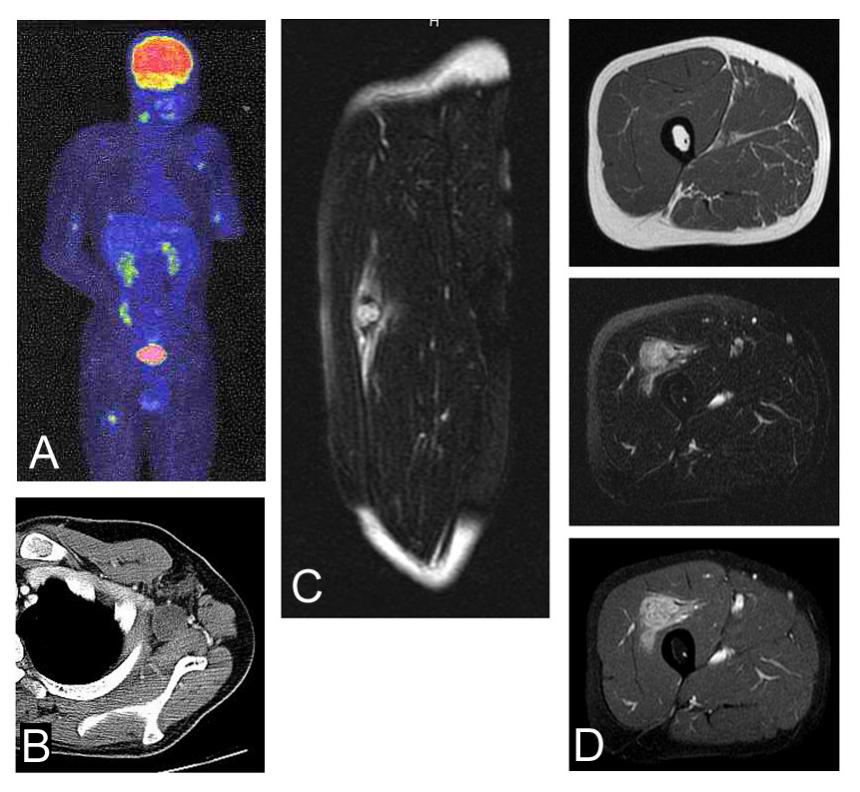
Metastatic lesions of epithelioid sarcoma. FDG-PET identifies lesions with an increased uptake in the right neck (SUVmax; 4.6), right upper arm (SUVmax; 4.1 g/ml), left upper arm (SUVmax; 4.2 g/ml), right thigh (SUVmax; 5.5 g/ml) left thigh (SUVmax; 2.0 g/ml), back (SUVmax; 3.6 g/ml), and lower back (SUVmax; 4.6 g/ml), as well as the left axilla (SUVmax; 3.9 g/ml) (arrows) (A). CT with contrast medium shows a swollen axillary lymph node (B). Sagittal MRI section of the right thigh shows a nodular lesion associated with prominent longitudinal abnormal signal intensity on T2-weighted image (C). The nodular lesion shows iso-intensity on T1-weighted fat-suppression image (top), and heterogeneous high-intensity on T2-weighted image (middle) (D). Enhancement with gadolinium can be seen on T1-weighted fat-suppression image (bottom) (D).
